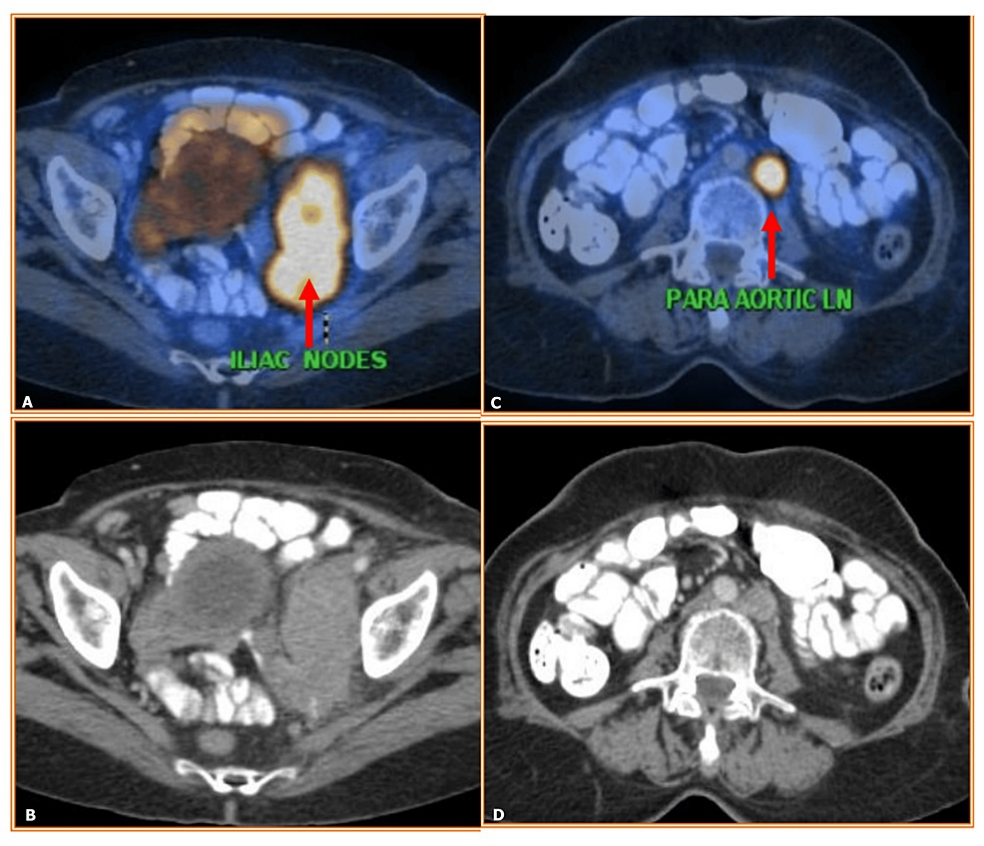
Pre-treatment PET-CT showing (A and B) multiple conglomerated iliac nodes with infiltration to iliac veins (52X42mm) SUVmax 13.6 and (C and D) enlarged left lower para-aortic nodes (16x14mm), SUVmax 12 (red arrows).
